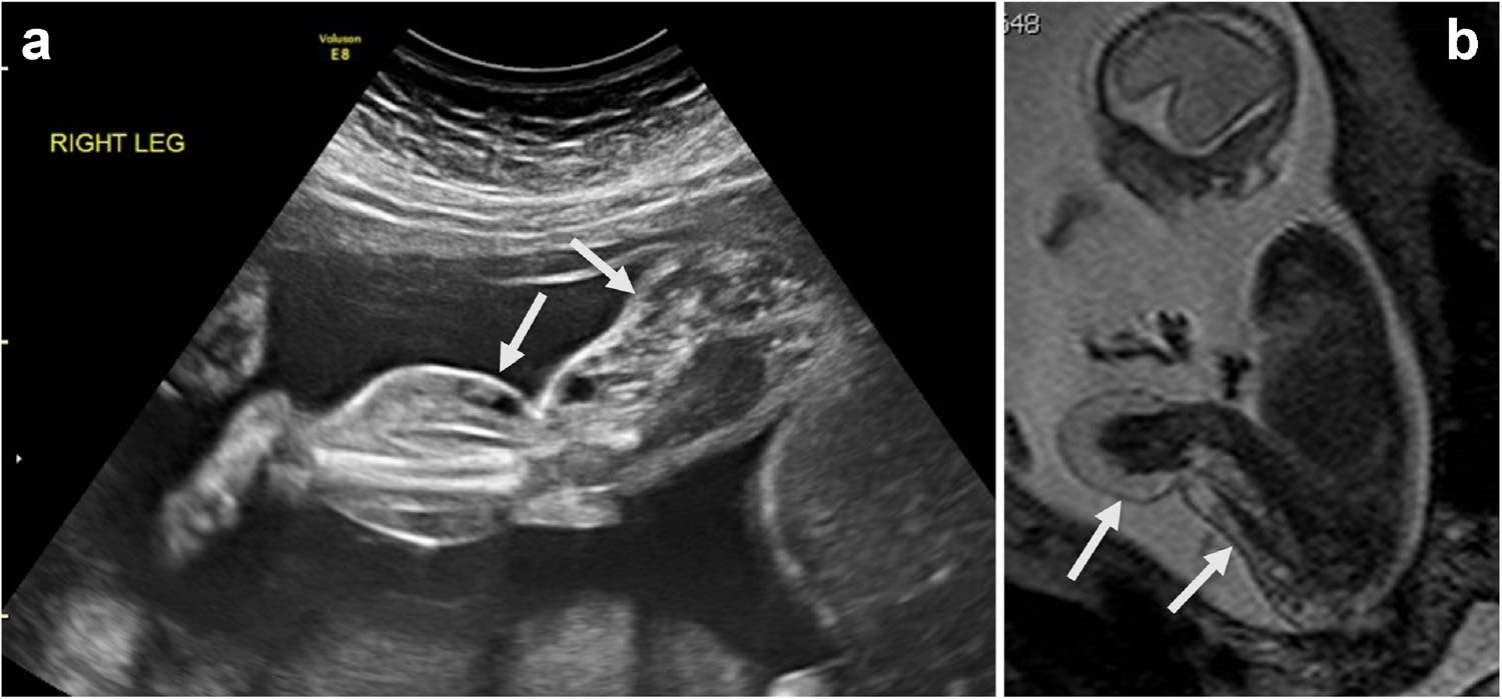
Venous malformation with overgrowth in a female fetus at 23-week gestational age. a, b Prenatal oblique ultrasound (a) and sagittal T2-weighted fetal magnetic resonance (b) images show multiple anechoic cystic and low-flow vascular structures and thickening of the subcutaneous fat (*arrows*), suggestive of a venous malformation with soft-tissue overgrowth. This fetus had genetically confirmed *PIK3CA* related overgrowth syndrome
